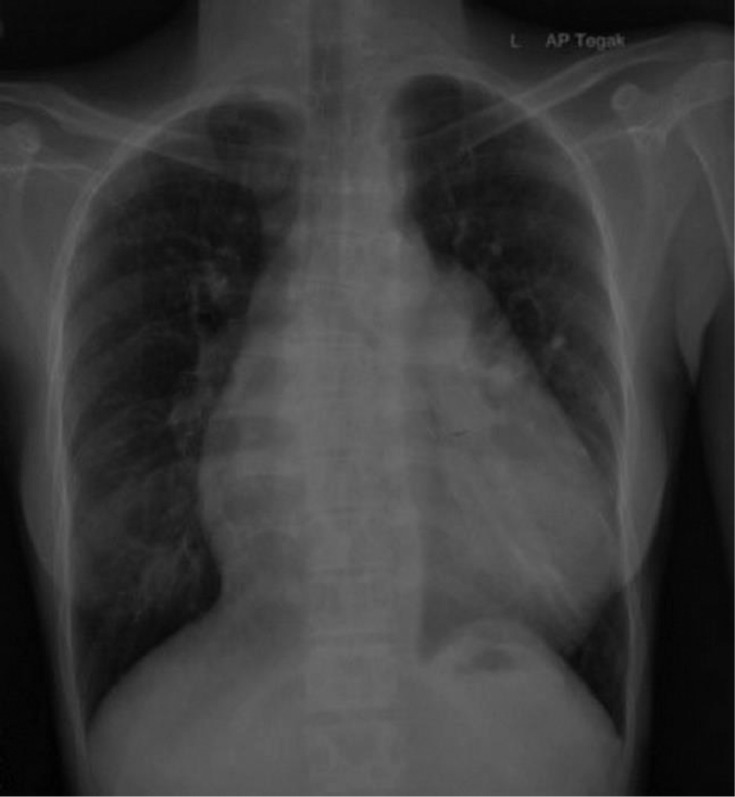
A chest radiograph revealed cardiomegaly without signs of pulmonary congestion, right bronchopneumonia.
